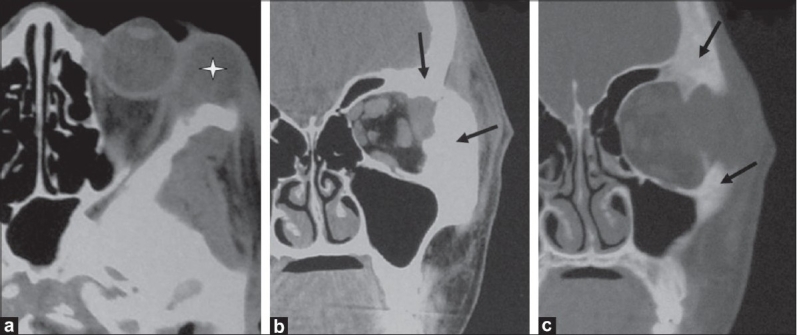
A 15-year-old boy presented with unilateral painless, nonpulsatile proptosis of the left eye. Axial contrast-enhanced CT scan (a) shows a left lacrimal abscess (asterisk). Coronal CT scans (b,c) show irregularity, destruction, thickening, and sclerosis of the adjacent frontal and zygomatic bones (arrow)
